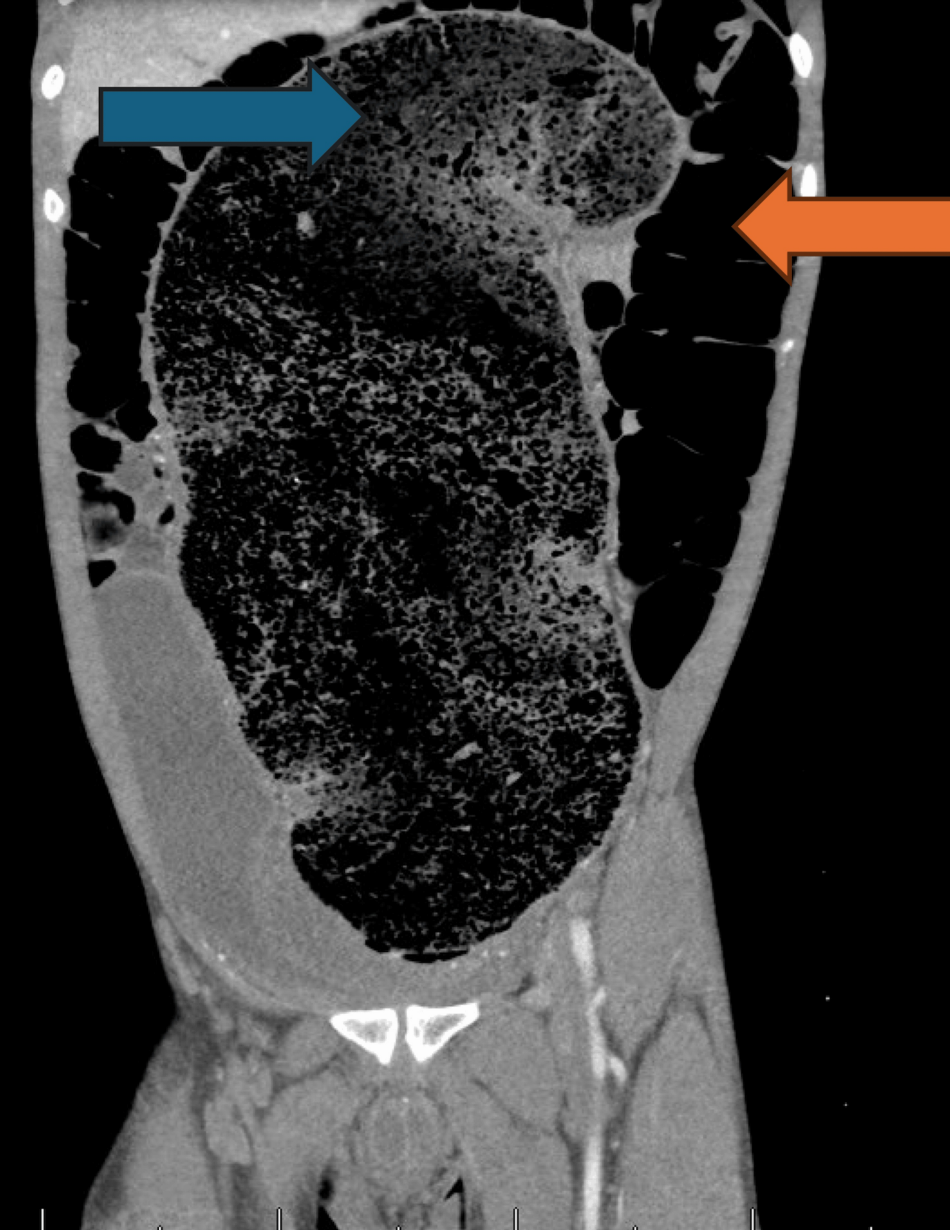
CT coronal view with gigantic fecaloma (blue arrow) and dilated loops of bowel (orange arrow)
